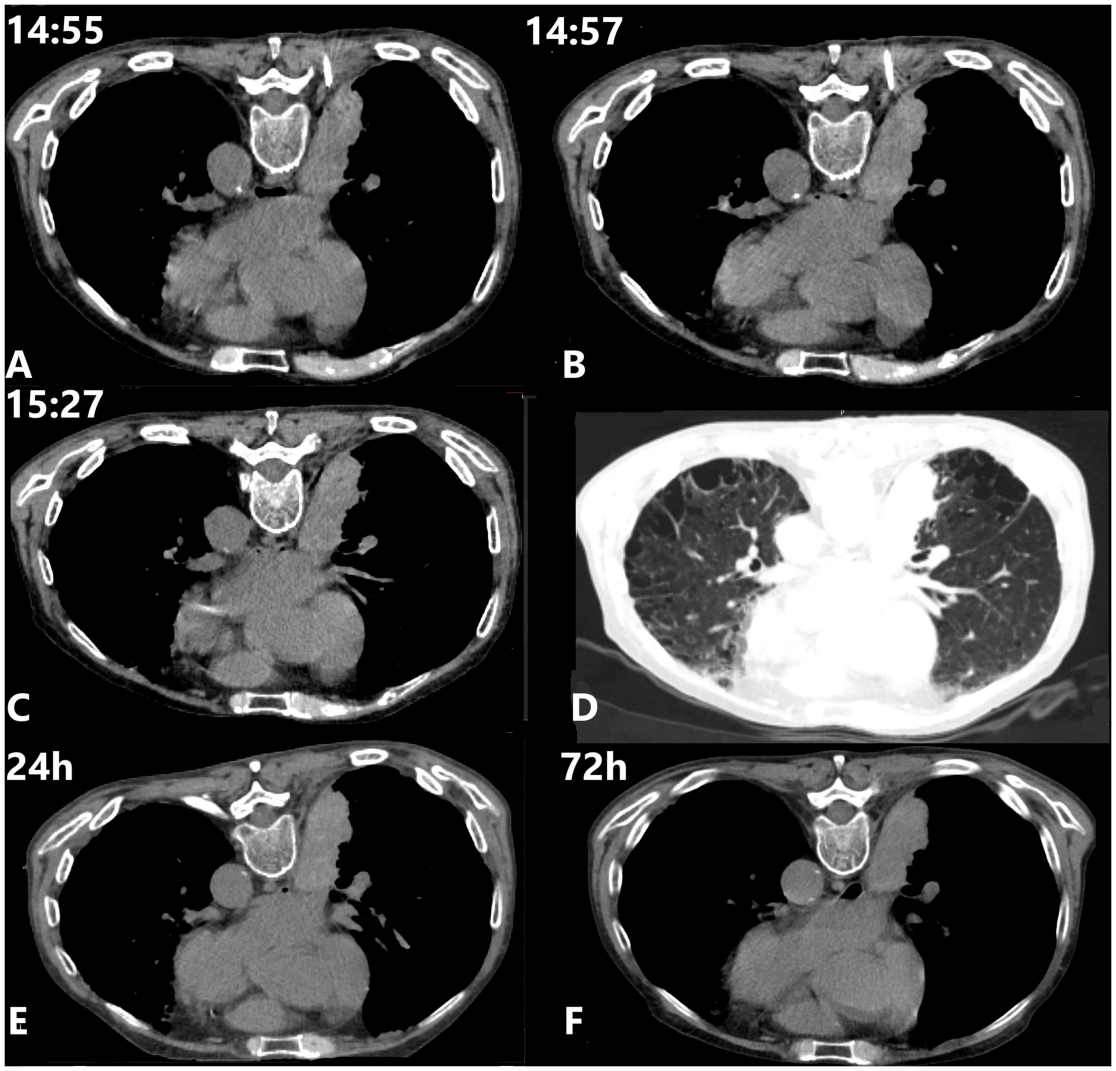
Intervention and evaluation. (A) Mechanical hemostasis was initiated. No visible hematoma or hemothorax was observed. The 5 mL syringe attached to the needle hub caused a slight tilt in the puncture needle. (B) No apparent signs of active bleeding were visible. (C,D) Following complete needle withdrawal, no apparent signs of active bleeding or pneumothorax were observed. (E,F) Follow-up CT scan images obtained at 24 and 72 h post-procedure.
